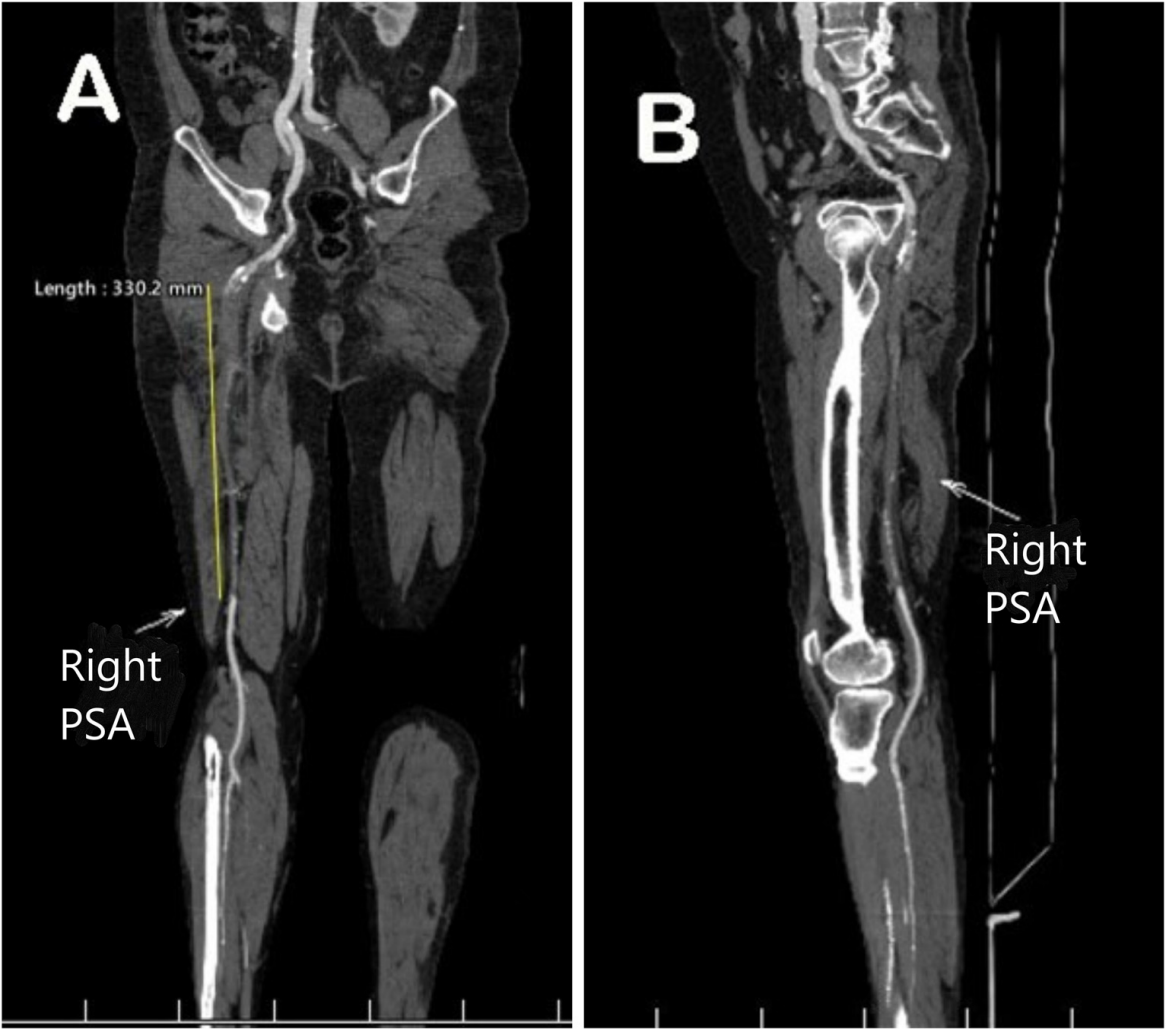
Coronal (A) and sagittal (B) CTA images of the right lower extremity. These images display the right PSA (arrows) with extensive intraluminal thrombosis measuring approximately 33 cm in length.
